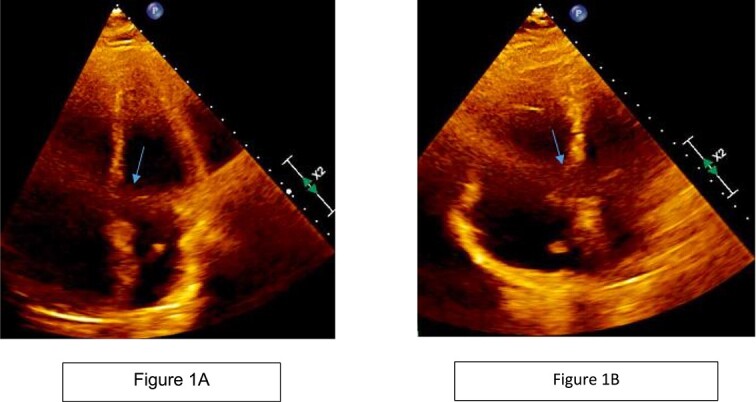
Transthoracic echocardiogram raising suspicion for a mobile mass in each atrium.
